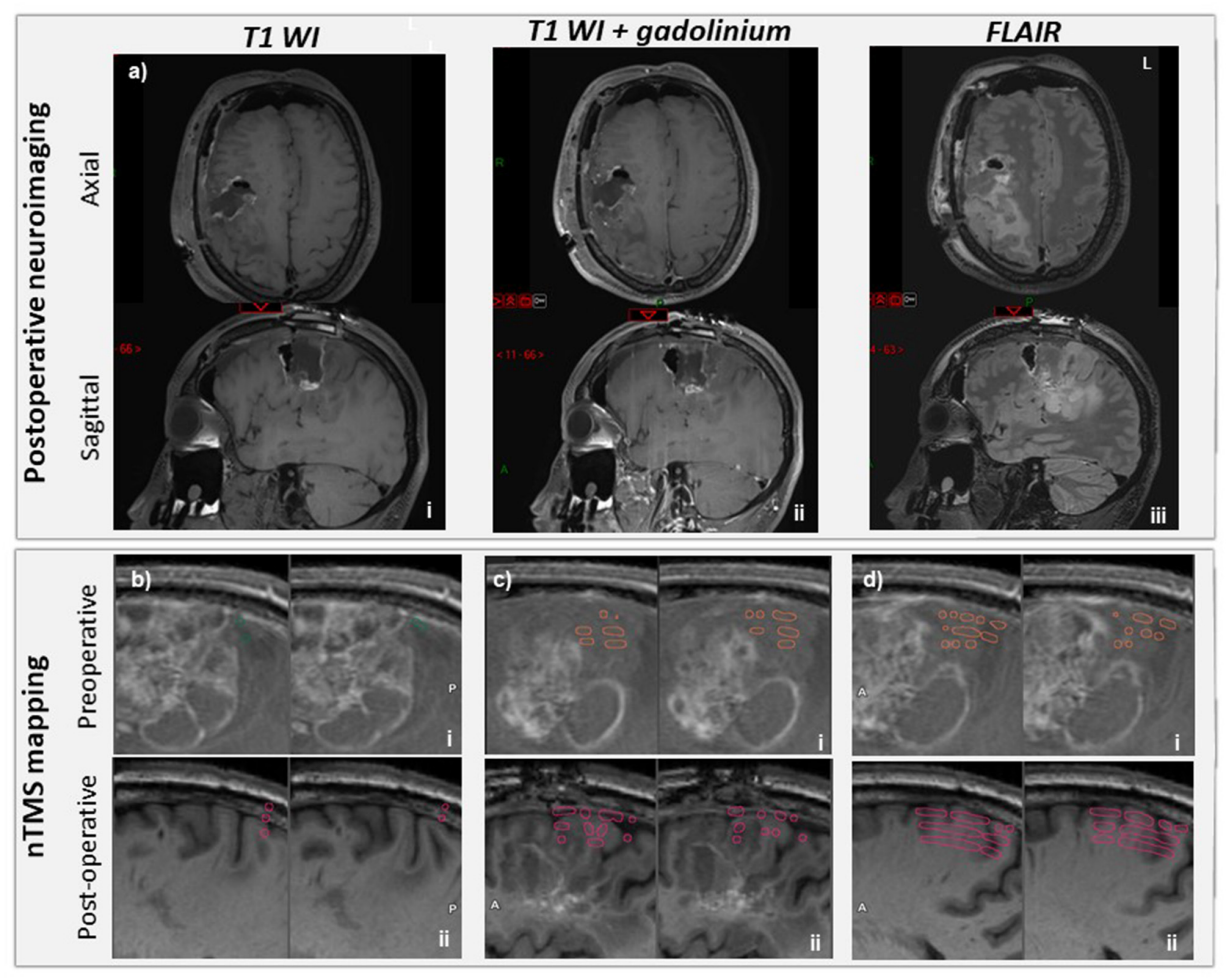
Post-operative neuroimaging status of the patient. (a) Day 1 postoperative MRI: axial (upper panel) and sagittal (lower panel) slices, T1WI (i), T1WI after gadolinium contrast injection (ii); FLAIR (iii). No residue with contrast enhancement was found. (b) Navigated transcranial magnetic stimulation (nTMS) mapping of left first dorsal interosseous (FDI) before (i, ROI in green) and 3 weeks after tumor resection (ii, ROI in pink). FDI nTMS map was shifted posteriorly 3 weeks after tumor resection. (c) nTMS map of left tibialis anterior (TA) before (i, orange) and 3 weeks after tumor resection (ii, pink) being larger with a posterior shift. (d) nTMS map of left deltoid before (i, orange) and 3 weeks after tumor resection (ii, pink) being larger with a posterior shift.
